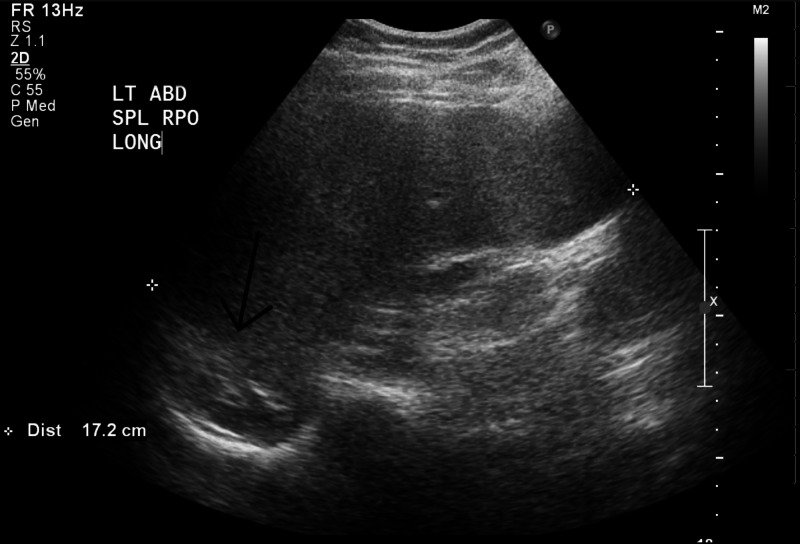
Long-axis gray-scale ultrasound image of the left upper quadrant in the right posterior oblique position demonstrates splenomegaly of up to 17 cm in the craniocaudal dimension Additionally, there is evidence of mass effect on the adjacent left kidney (black arrow).
